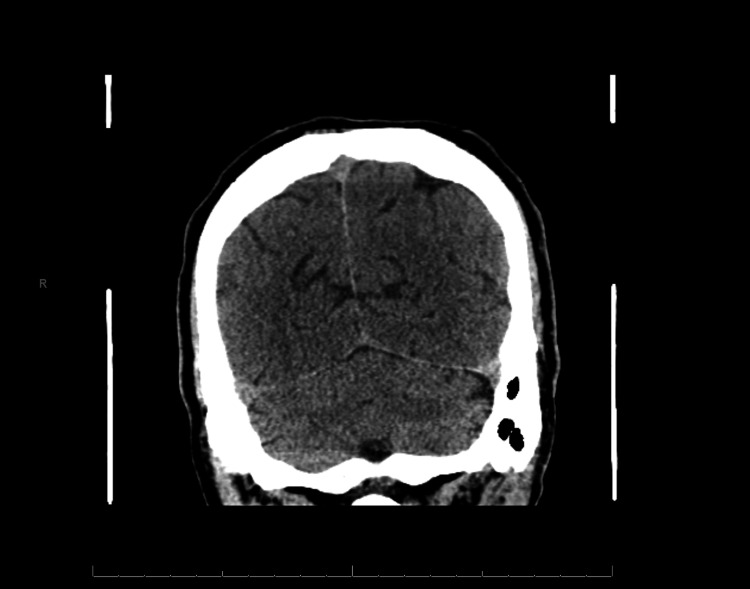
Non-contrasted head computed tomography demonstrating only senescent changes with cortical atrophy appropriate for age.
